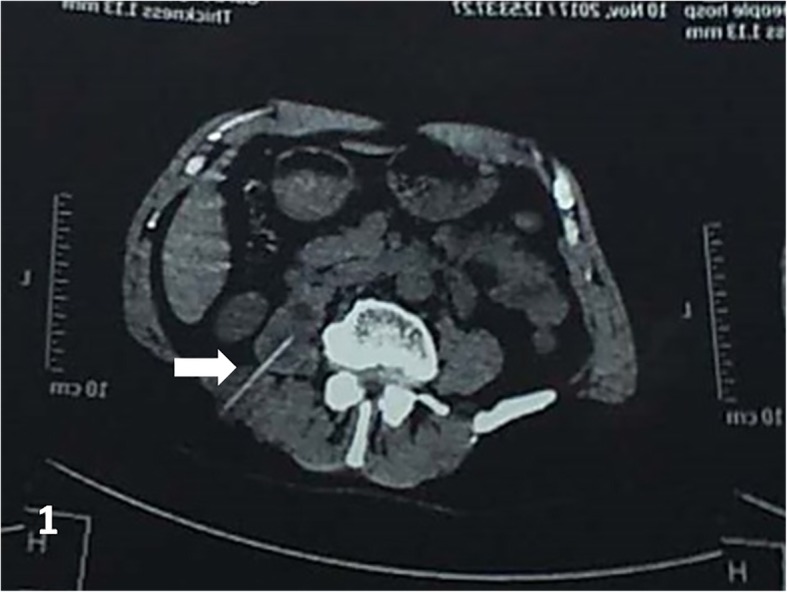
Computed tomography of the abdomen revealed a hyperdense foreign body traversed across the right psoas and sacrospinalis muscle (arrow)
